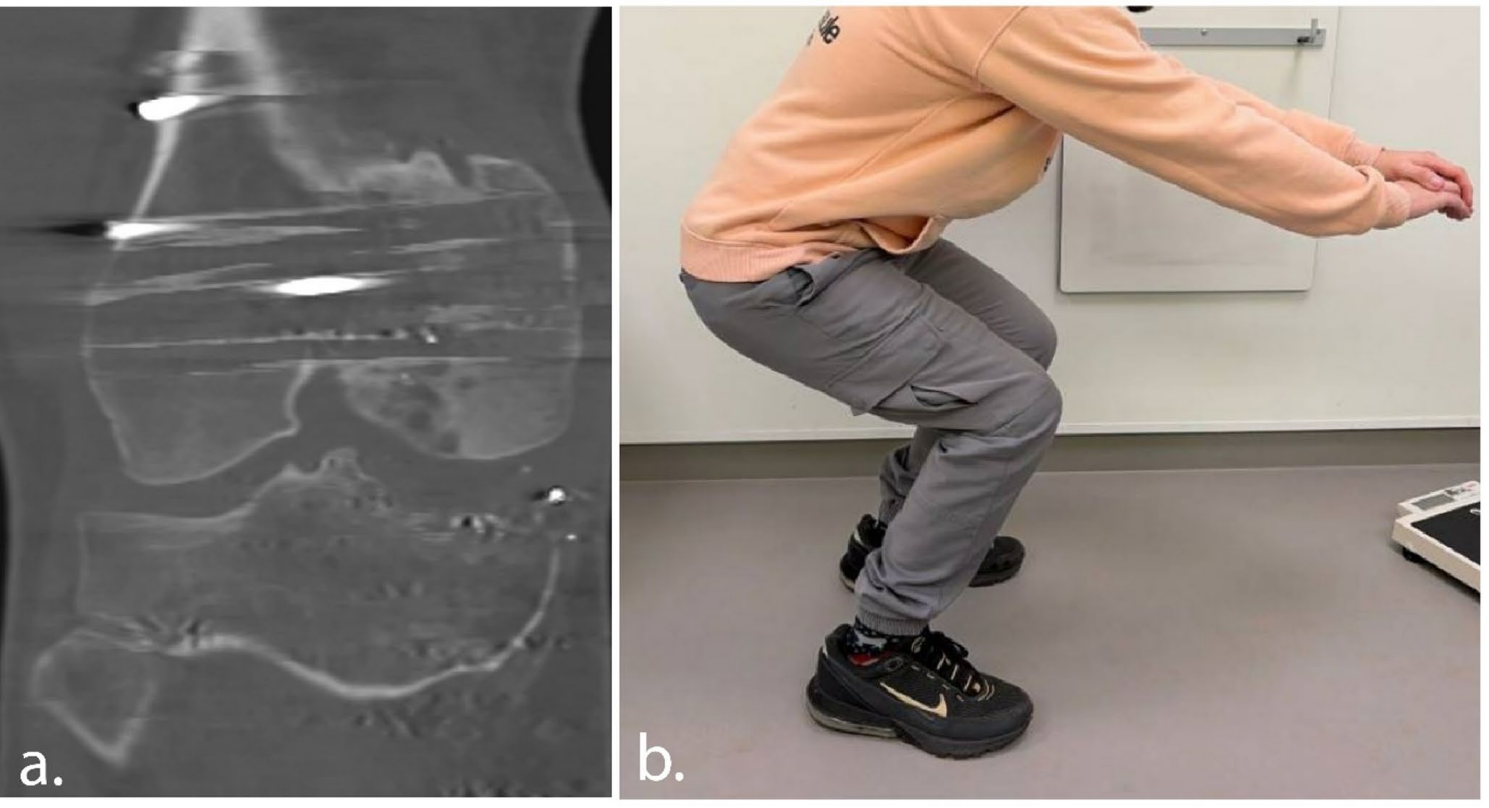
At 15 months follow-up, the patient showed favourable clinical recovery, with the CT-scan of the medial allograft demonstrating good integration (a). Due to recurrent medial valgus instability a revision MCL reconstruction was performed. After the final follow-up at three years good functional outcome and squatting ability was observed (b)
